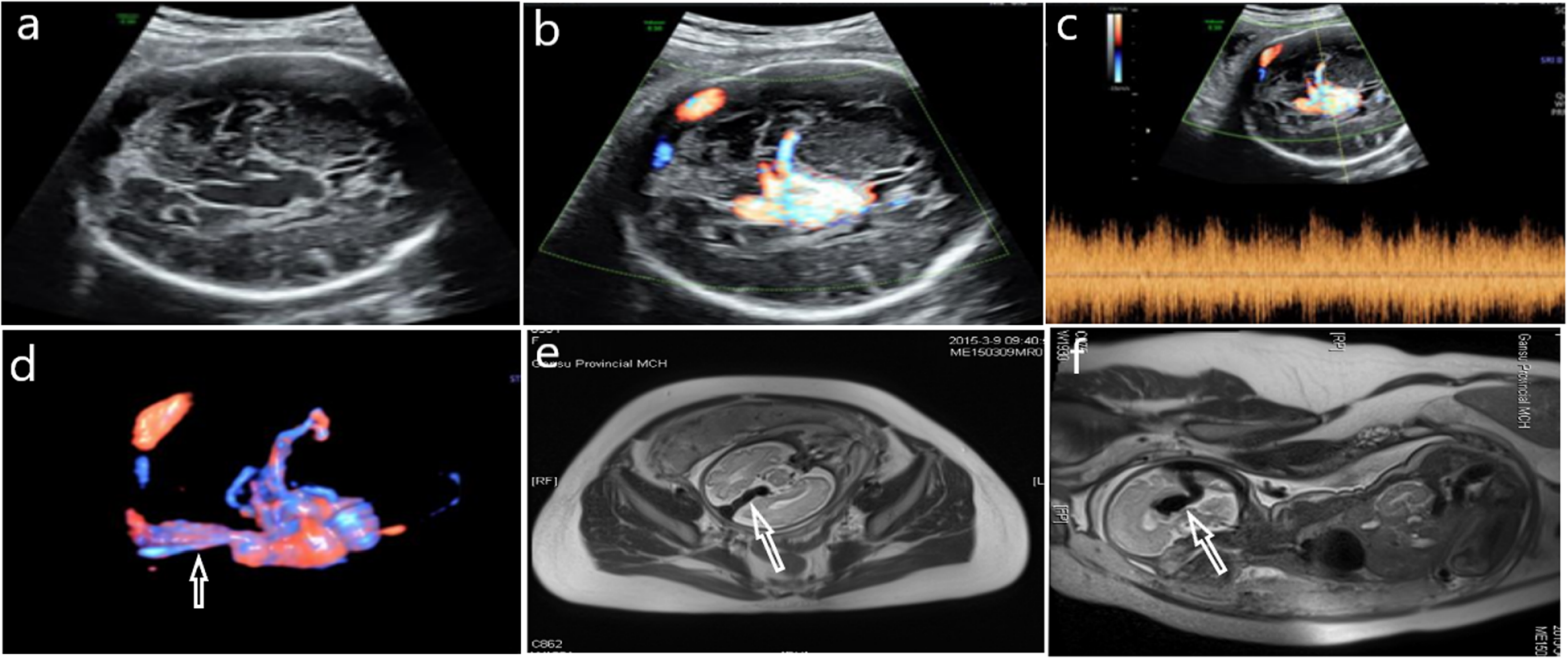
Case 2, 30-week prenatal ultrasound and MRI images of a VGAM foetus. a: Two-dimensional ultrasound shows a huge thin-walled echoless area next to the midline of the brain. b: Colour Doppler shows a full blood-flow signal in the brain’s echoless area. c: The skull’s echoless area shows arteriovenous leak with arteriovenous mixed spectrum; d: The reconstructed three-dimensional energy Doppler image clearly shows the dilated VGAM, and obvious straight sinus is displayed (white arrow). e: MRI cross-section of the foetal skull showing the dilated VGAM through flow phenomenon (shown by white arrow). f: Sagittal section of the foetal brain showing the dilated Galen’s venous aneurysm by flow phenomenon (white arrow)
